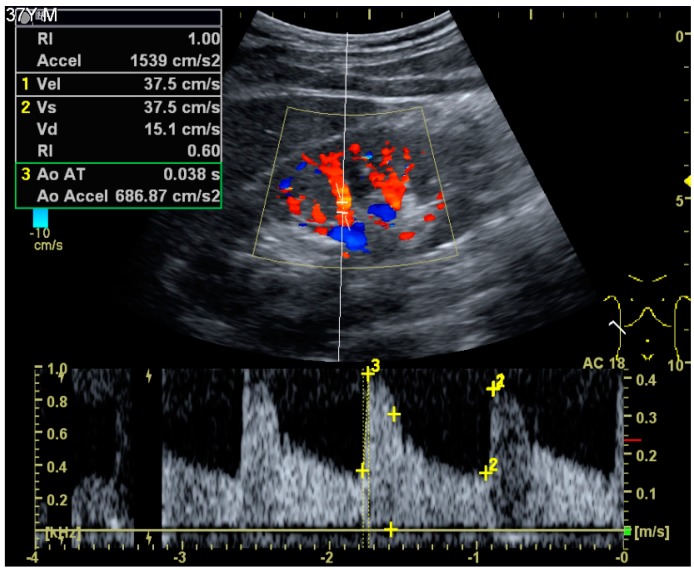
Doppler ultrasound (US) of a normal adult kidney with the estimation of the systolic velocity (Vs), the diastolic velocity (Vd), acceleration time (AoAT), systolic acceleration (Ao Accel) and resistive index (RI). Red and blue colors in the color box represent flow towards and away from the transducer, respectively. The specrogram below the B-mode image shows flow velocity (m/s) against time (s) obtained within the range gate. The small flash icons on the spectrogram represent initiation of the flow measurement.
